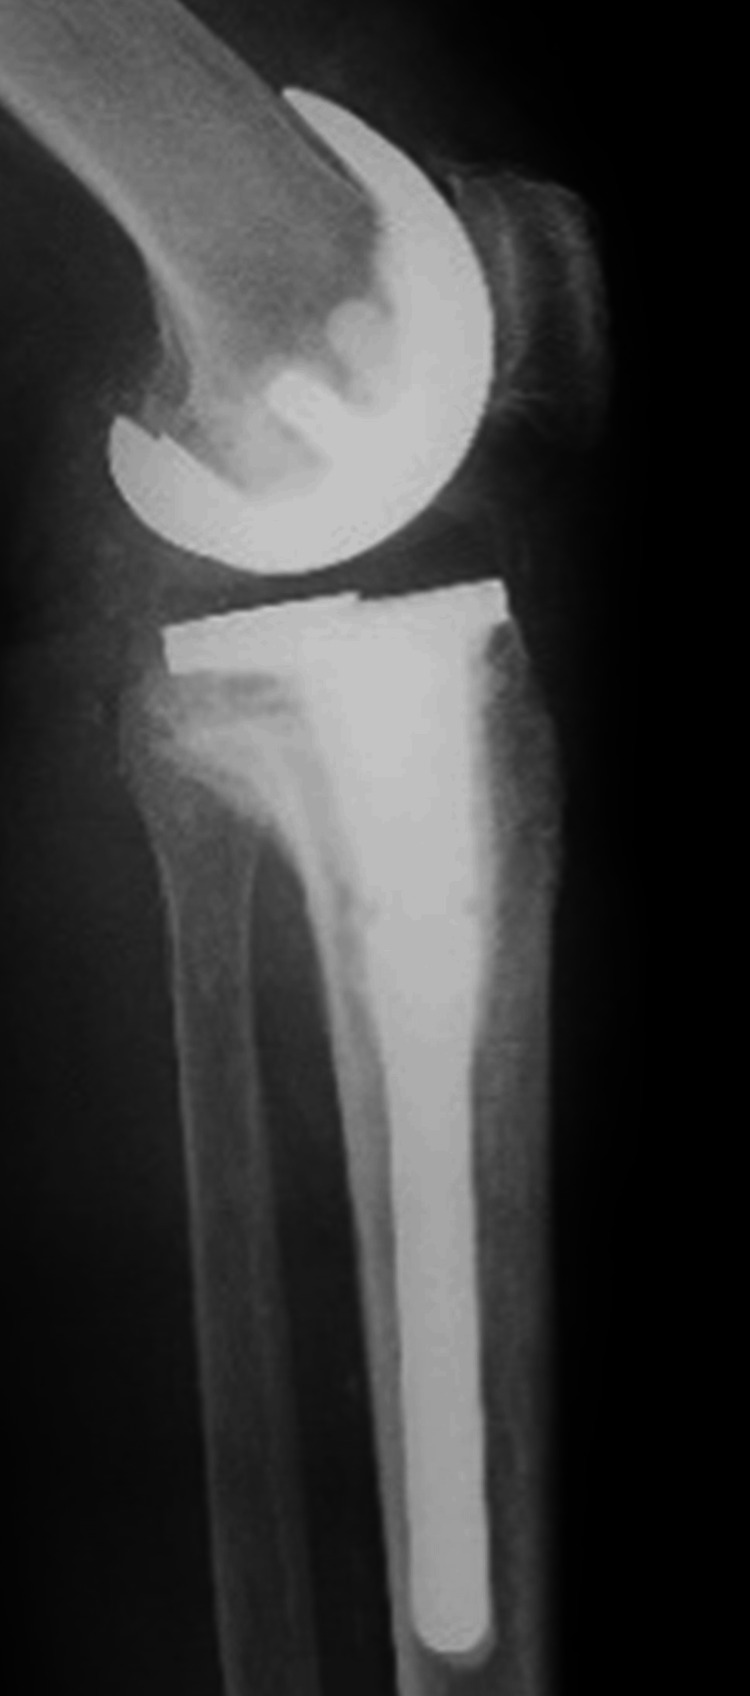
Radiography after conversion to TKA (lateral view) TKA: total knee arthroplasty.
